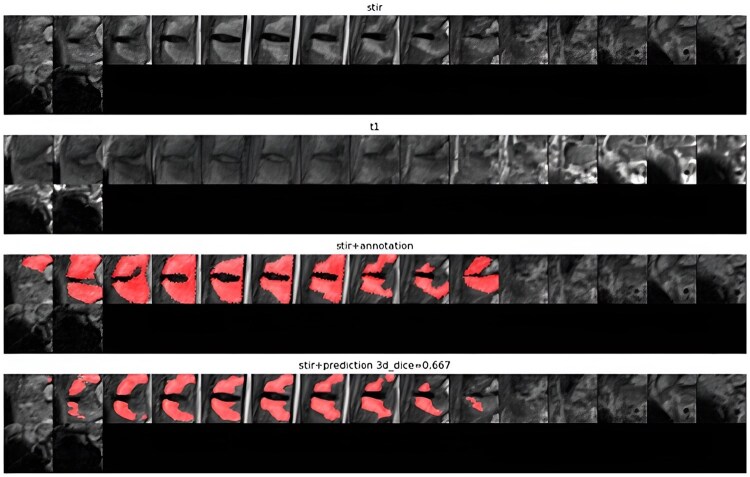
Quantitative examples of MEASURE 1, including both the annotation by the expert radiologist and the model annotation prediction. First panel, STIR sequence; second panel, T1-weighted sequence; third panel, annotation by the expert in the STIR sequence; fourth panel, model annotation prediction in the STIR sequence
